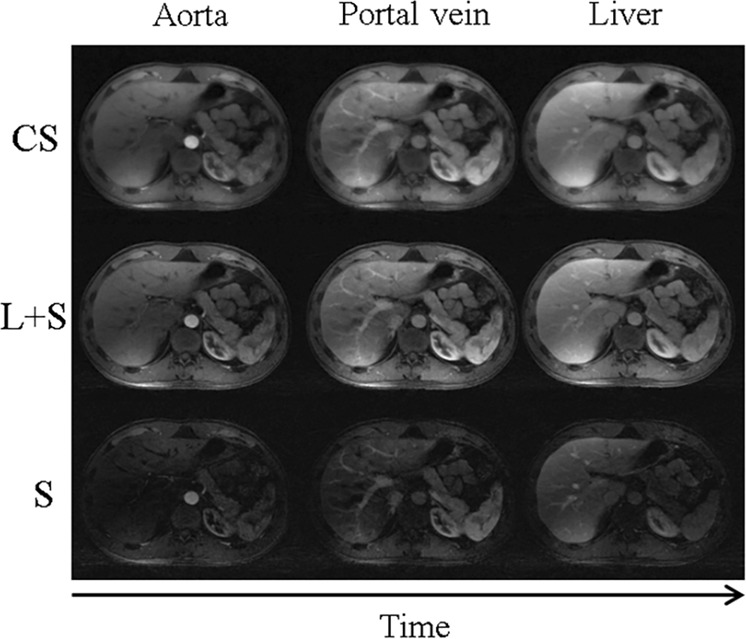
Data matrix decomposition for accelerated dynamic MRI of three contrast enhanced image phases.^18^ Compressed sensing (CS) images reflect sparsity only, while low rank plus sparse (L + S) images provide improved spatiotemporal resolution resulting from the automated background suppression of the sparse (S) components, enhancing contrast resolution. (Reproduced with the permission of the Institute of Electrical and Electronics Engineers)
